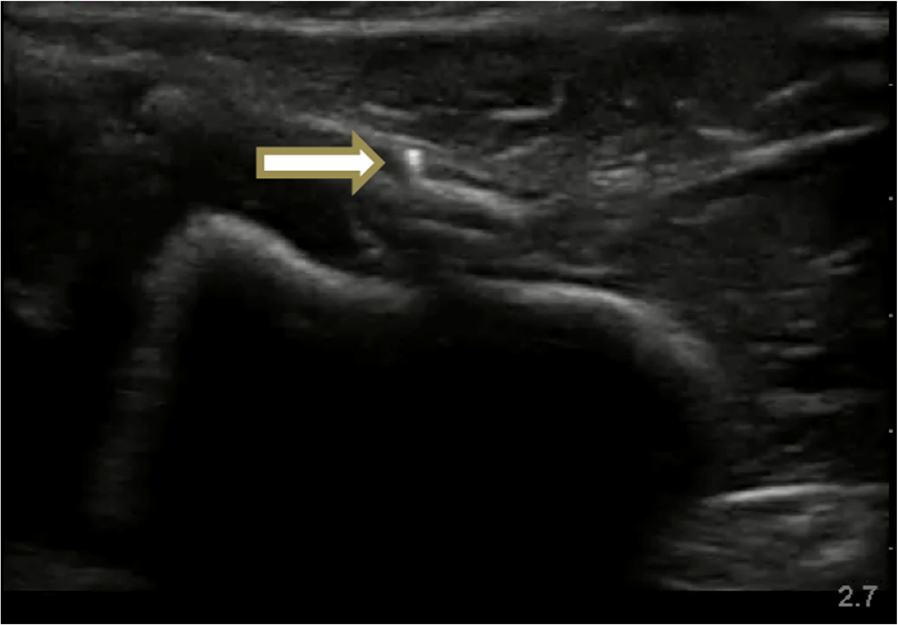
Metal foreign body (arrow) as seen by ultrasound.
